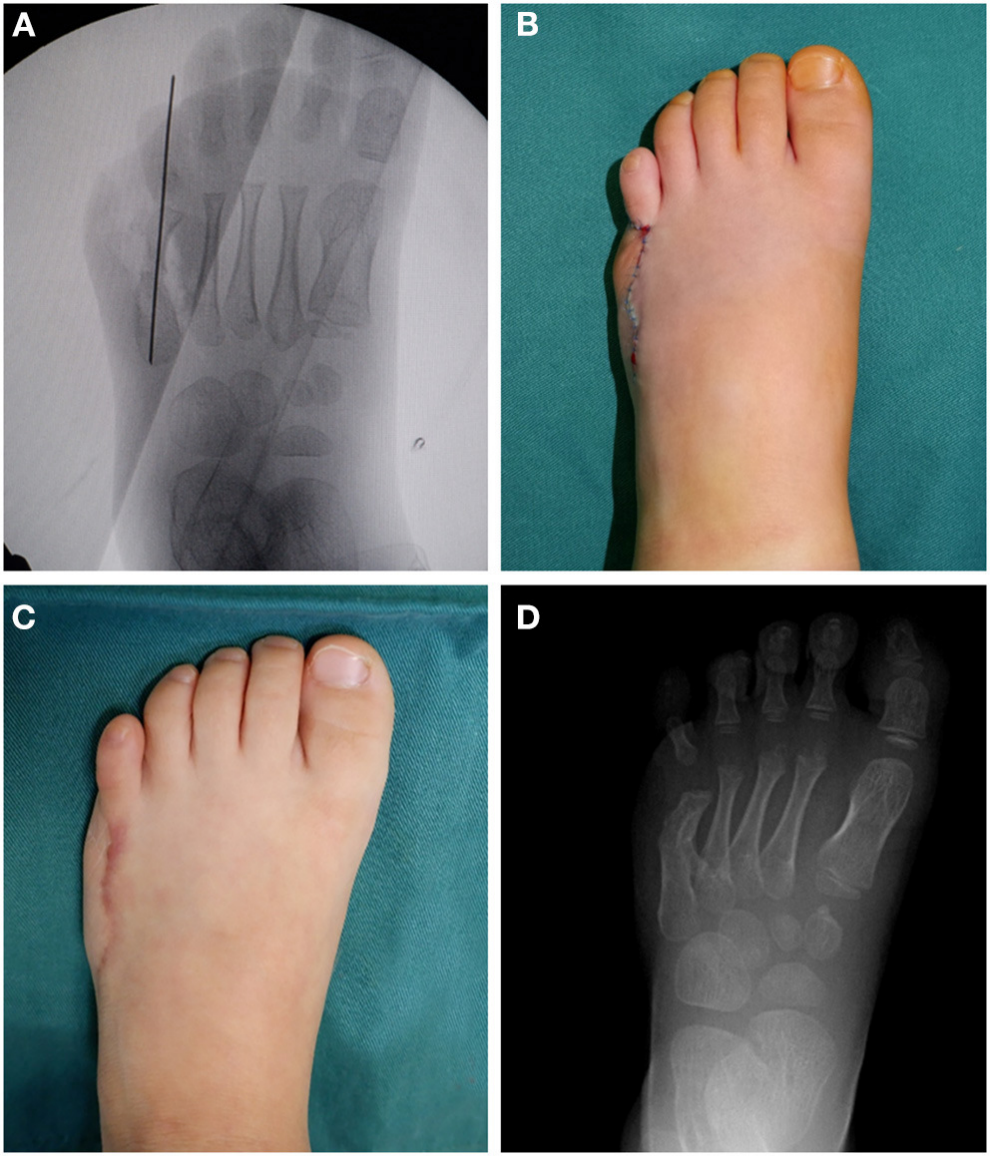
Intraoperative fluoroscopic images, immediate postoperative appearance and 1-year follow-up findings. (A) Intraoperative fluoroscopic radiographic images showing the fifth ray axis was restored and the wide forefoot was improved. (B) Immediate postoperative photos showing the appearance of the foot. (C) Photos showing the appearance of the operated foot at 1-year after surgery. (D) Radiograph showing good healing ang the fifth ray axis was restored, taken 1-year after surgery.
